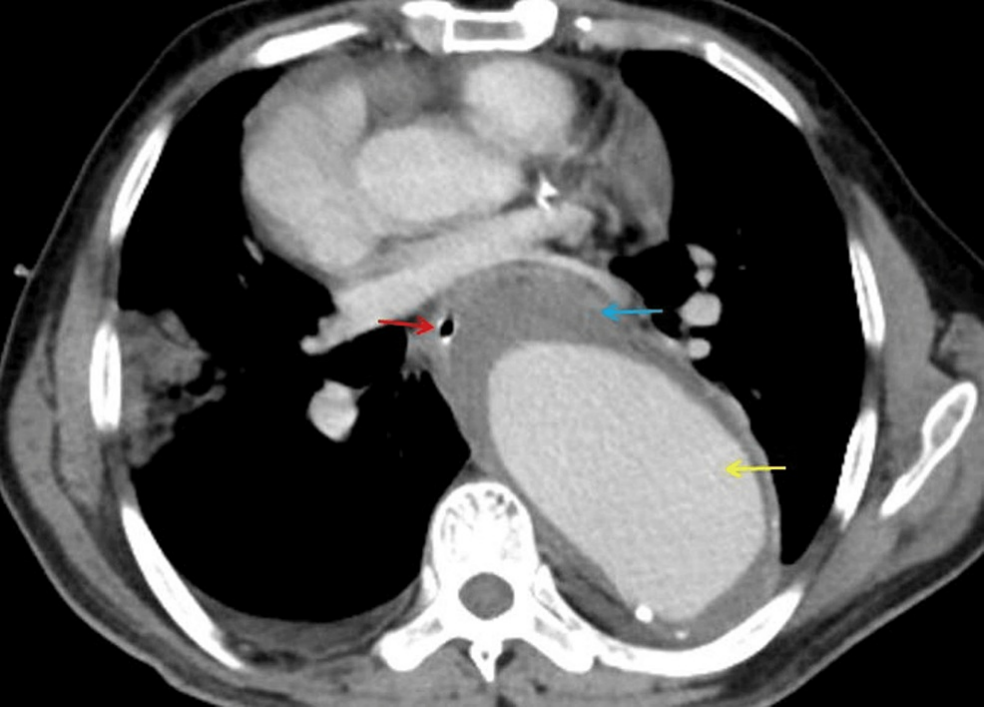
Computed tomography (axial view) of the chest Computed tomography (axial view) of the chest showing descending aortic aneurysm (yellow arrow), thrombus within the aneurysm (blue arrow) with the nasogastric tube within the esophagus (red arrow).
